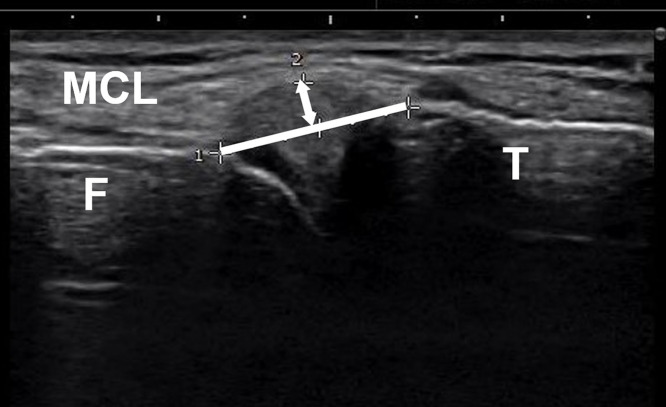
US measurement of medial meniscus extrusion MME. MME = medial meniscus extrusion. US = ultrasonography. F = femur. T = tibia. MCL = medial collateral ligament. ↕ = MME distance.
